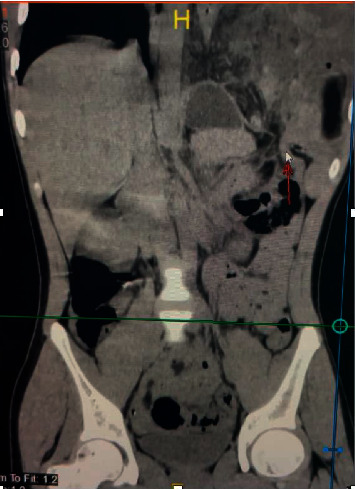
The thoracoabdominal CT scan without contrast showing a left-sided diaphragmatic defect (indicated by the white arrow) with herniation of the bowel into the thorax cavity (coronal view).
